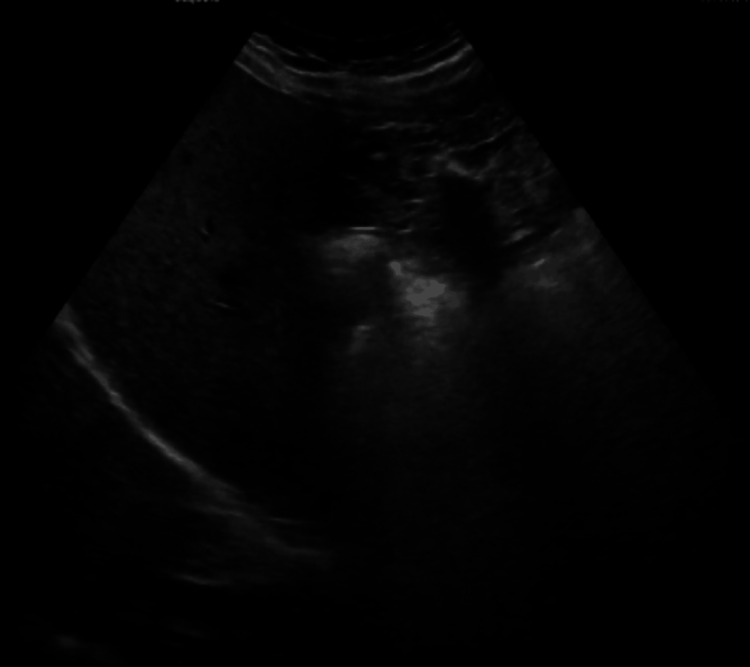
Abdominal ultrasound demonstrating post-surgical changes and no biliary obstruction
